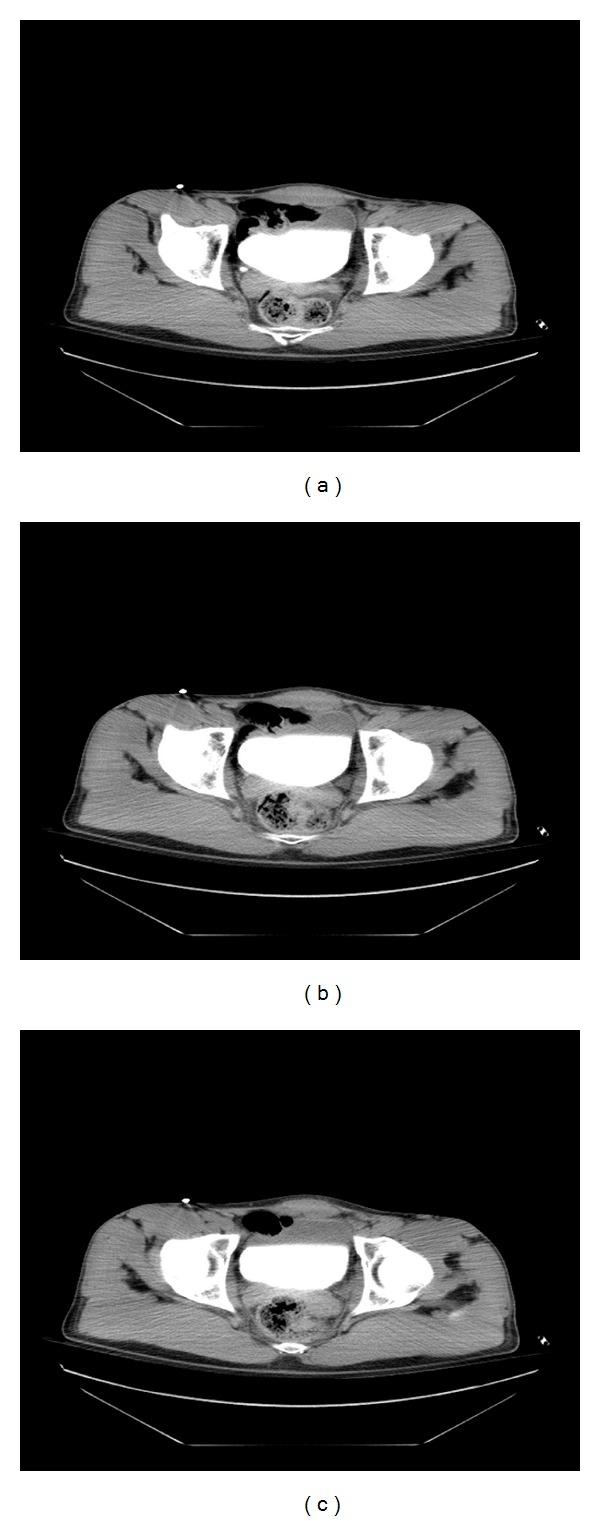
Contrast-enhanced CT scan (Siemiens). Contrast fills bladder without outflow. Urethra has not been revealed on these scans.
